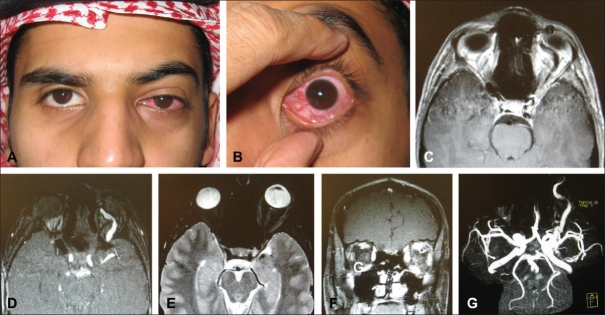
This 17-year-old male presented with sudden development of decreased vision in the left eye, proptosis, conjunctival chemosis, ptosis and elevated intraocular pressure 1 year after having experienced head trauma (A, B). Imaging studies revealed classic features of CCF on the left side manifested as evidence of the enlargement of the superior ophthalmic vein (SPV)(C). Dilated signal-void serpiginous structures are seen intraconally and extending to the left cavernous sinus (C, D). In addition, there were enlarged extraocular muscles on the left side, as evidenced by axial and cornonal MRI (E, F). MRA and MRV confirmed the diagnosis of CCF with markedly enlarged left SOV (G)
